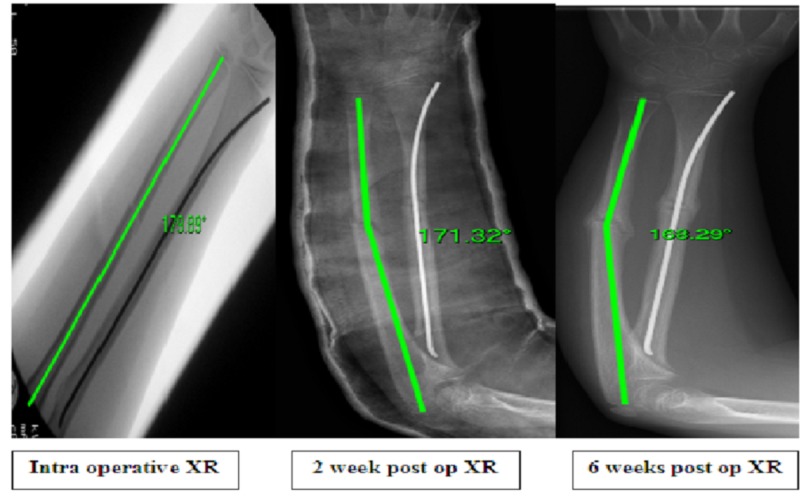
Intraoperative, 2 week and 6 week radiographs of patient with S-EIN of radius and postoperative deformity of the ulna. Shown with on-screen angle measurement. S-EIN - Single elastic intramedullary nail
